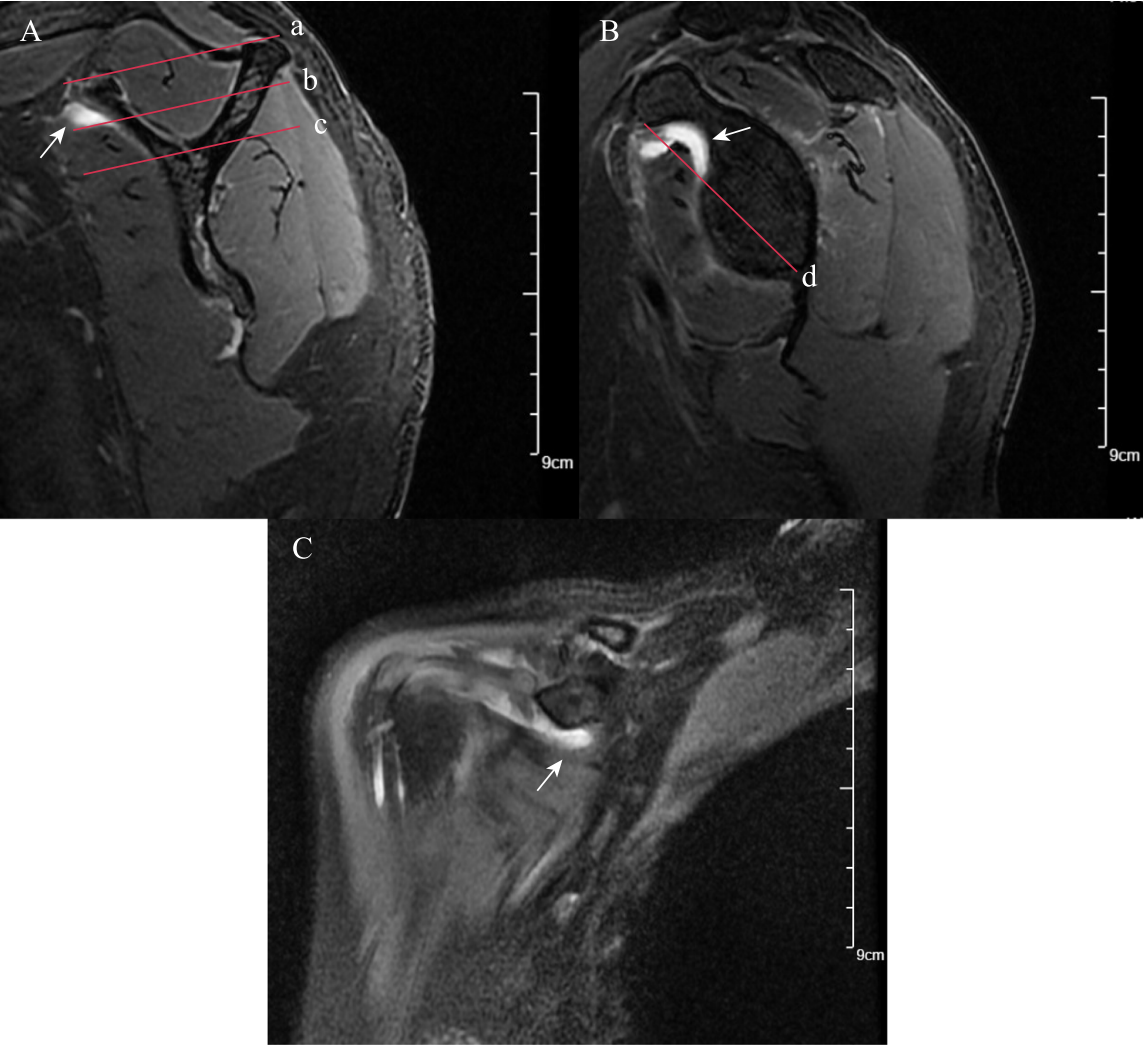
MRI images of subscapular muscle atrophy, fluid accumulation, and fluid area ratio (en-face). A SSC muscle atrophy classification on Y- face plane. Parallel line intercepting supraspinatus fossa opening (red solid line a), and parallel line (red solid line b) bisecting the perpendicular distance between line (red solid line a) and line (red solid line c). Based on these lines, SSC position was classified. Between line (a) and (b) was classified as grade (I), between line (b) and (c) was classified as grade (II), below line (c) was classified as grade (III). Fluid accumulation in the Y-face plane (white arrow). B En-face plane showing base to-tip line (BTL): the inferior pole of glenoid to coracoid tip on en-face (red solid line d). Using BTL, muscle atrophy of SSC is classified as grade I (tendon and muscle exist above the BTL), grade II (only tendon exist above the BTL), and grade III (tendon and muscle exist below the BTL). The fluid area ratio was the ratio of the effusion area to the area surrounded by the coracoid process, glenoid, and BTL in the en-face. Fluid accumulation in the en-face plane (white arrow). C Fluid accumulation in the coronal plane (white arrow)
